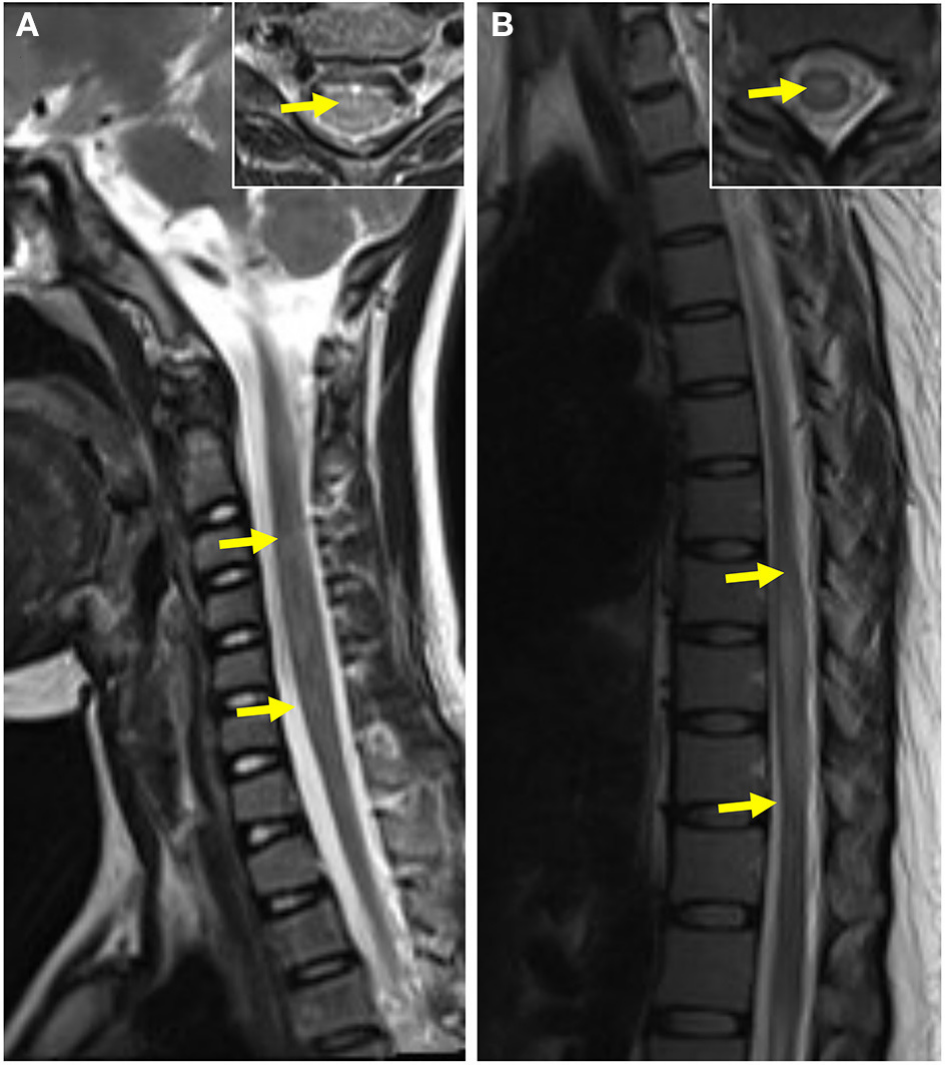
Spinal cord MRI of pediatric and adult patients. (A) T2-weighted MRI of the spinal cord of a pediatric patient showed abnormal patchy signals at the cervical segment The coronal image of lesion-involved slices was showed in the right box. (B) T2-weighted MRI of the spinal cord of an adult patient showed a striped hyperintense lesion at the thoracic segment. The coronal image of lesion-involved slices was showed in the right box.
